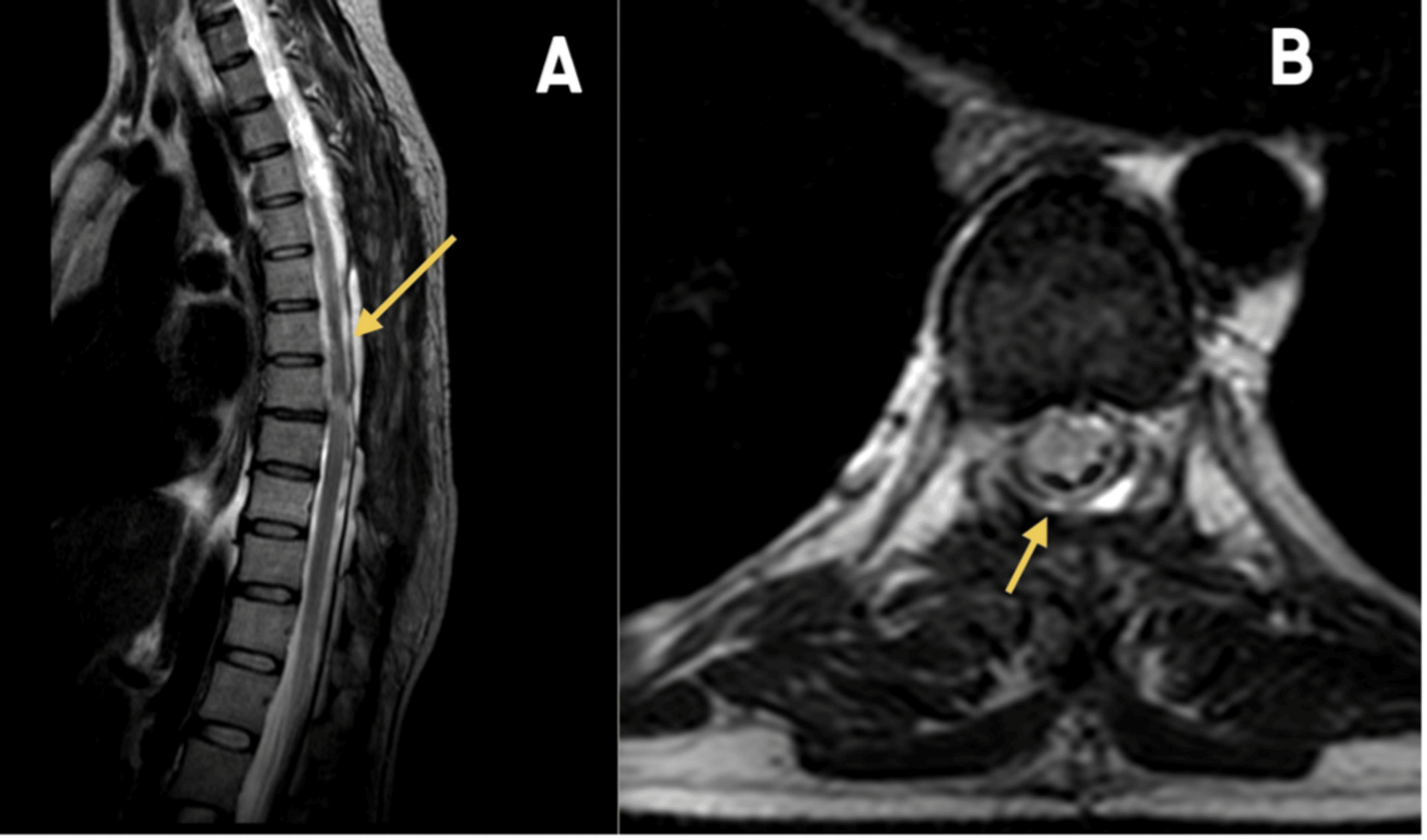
Postoperative images (A) Sagittal T2-weighted image showing complete excision. (B) Axial T2-weighted image.
